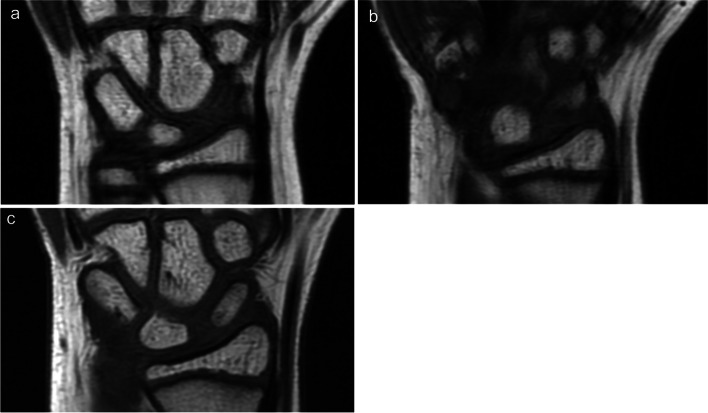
Temporal changes in MRI T1-weighted images. At 1 year after the initial visit, scaphoid bone still showed low intensity (a), though partial recovery of intensity change was observed only in the distal pole (b). At 2 years after the initial visit, normal intensity on the whole scaphoid bone was observed (c)
